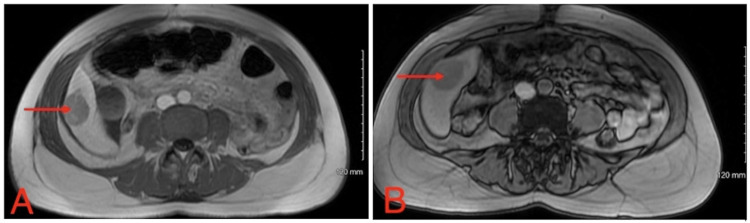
MRI abdomen (A) MRI abdomen three days postoperative. Red arrow identifying a 3.8 cm mass of the right liver consistent with HA. (B) MRI abdomen three months postoperative, with the red arrow depicting a 3.8 cm right liver lesion. MRI: magnetic resonance imaging, HA: hepatic adenoma
